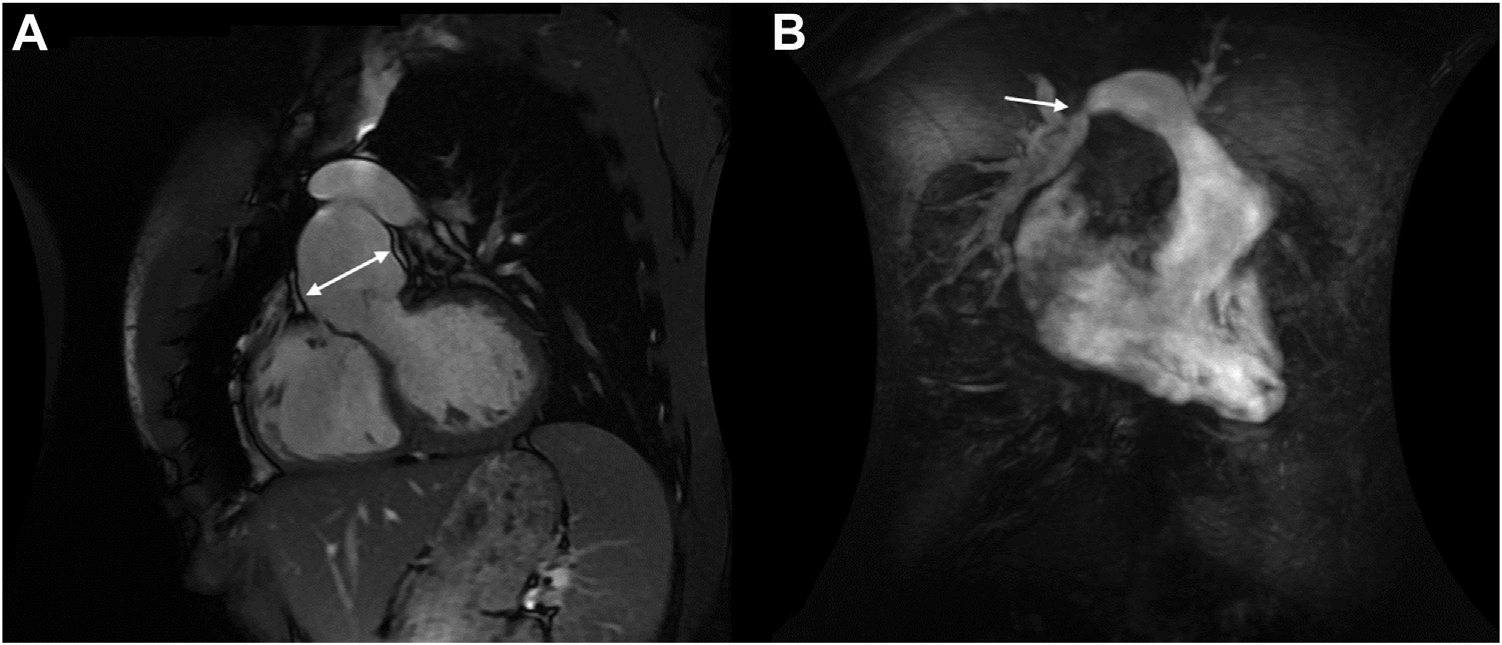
Cardiac magnetic resonance imaging. (A) Demonstration of the left-ventricular outflow tract with severe dilatation of the aortic root. (B) Severely enlarged right ventricle with the pulmonary artery and branches. The arrow identifies the area of right pulmonary artery stenosis.
